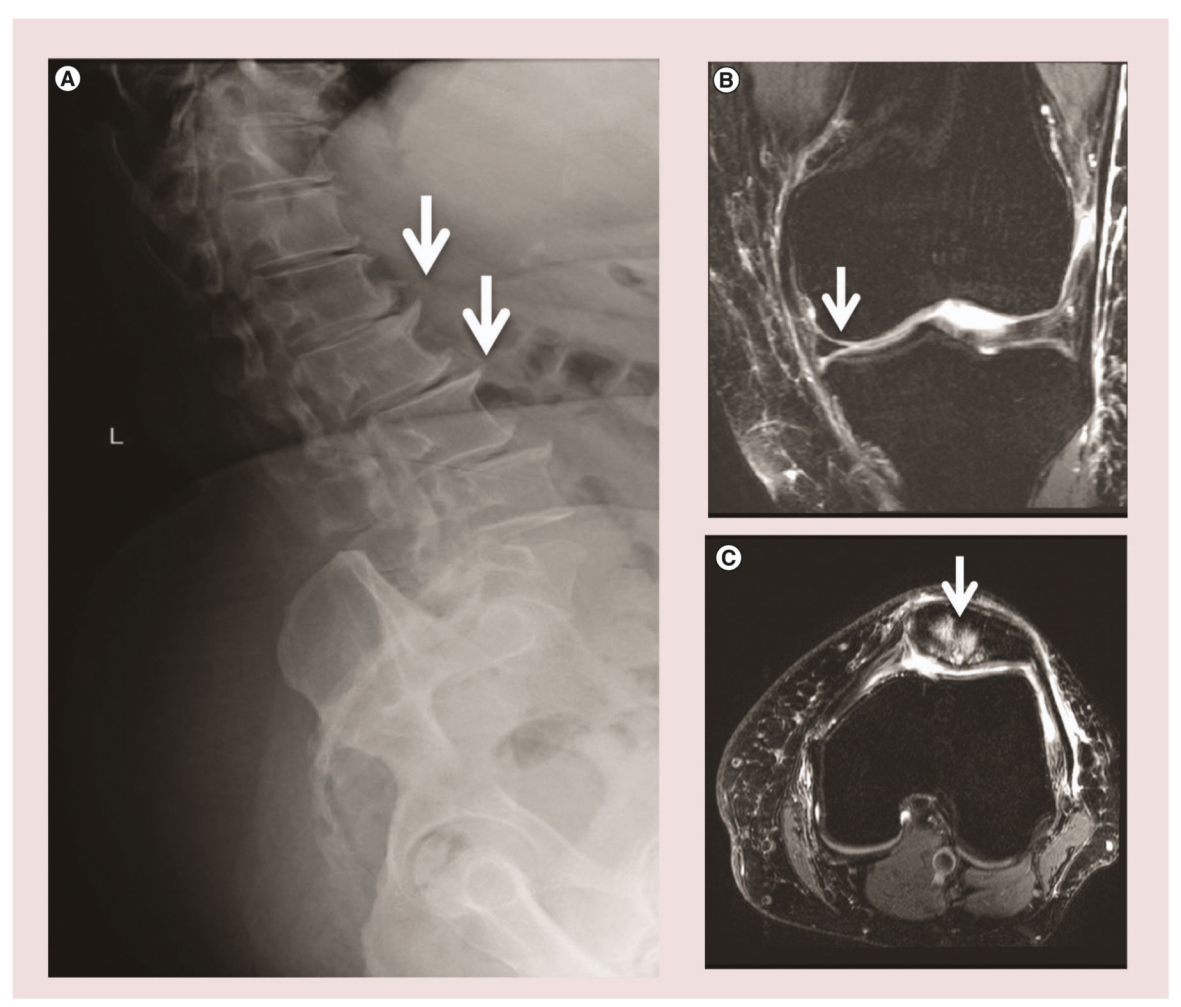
Radiographic features of tissue damage in osteoarthritis (A) Example of osteophytes (white arrows) shown in the anterior lumbar vertebral bodies. (B) MRI with T2-weighted sequences demonstrating cartilage loss (white arrow) in patient with osteoarthritis. (C) MRI with T2-weighted sequences demonstrating bone marrow lesions localized to the knee patella (white arrow) in a patient with osteoarthritis. Image acquisition paradigm for MRIs courtesy of Franklyn Howe (St George’s University, London, UK).
